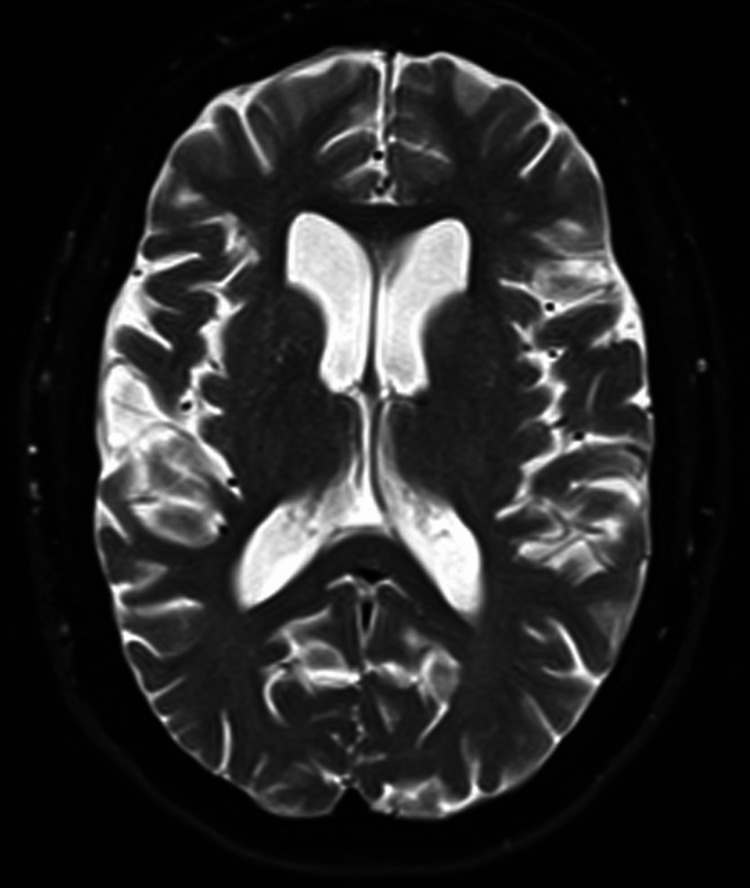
MRI showing generalized age-appropriate brain atrophy as evidenced by sulcal widening throughout the brain.
